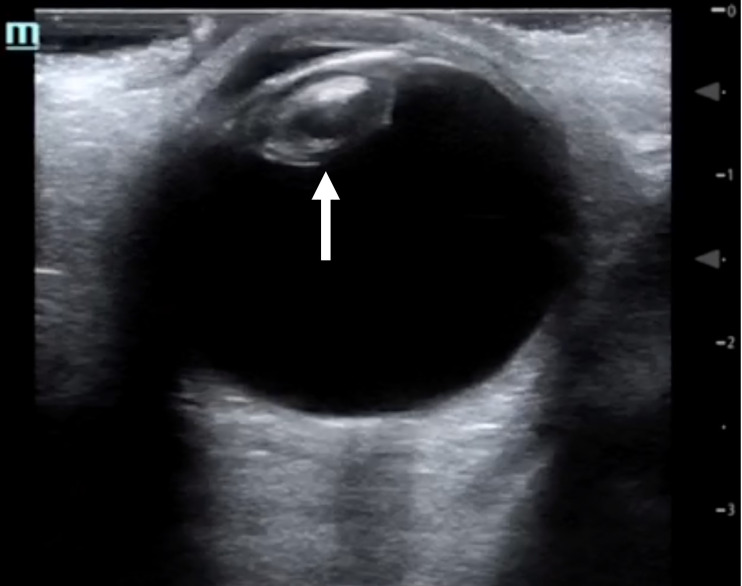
Point-of-care ocular ultrasound image in the transverse plane, revealing lens calcification consistent with cataract (arrow).
